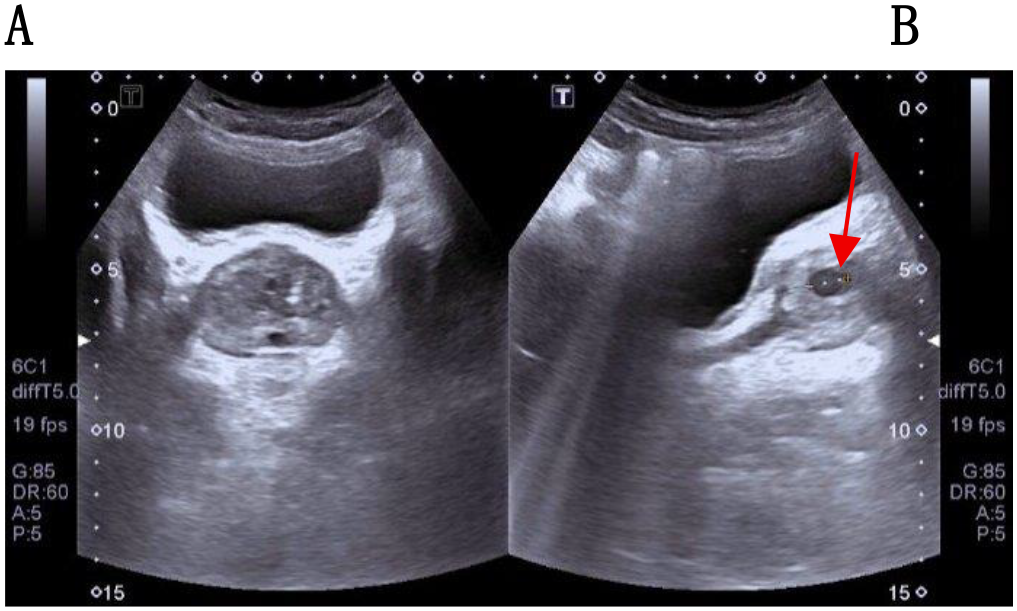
The patient’s urinary system ultrasound images. (A) The inner gland of the prostate is enlarged, and multiple hyperechoic foci are visible in the inner gland. (B) A relatively large cystic echo is seen within the prostate gland.
